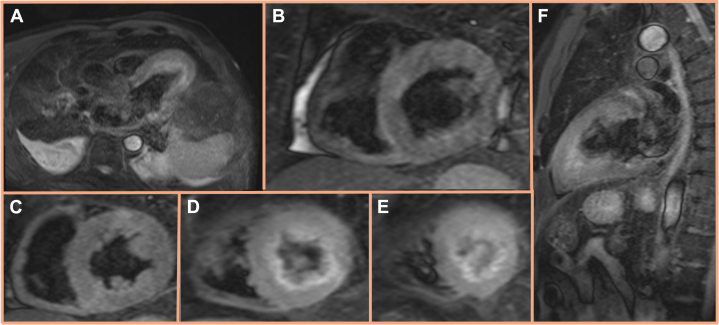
Cardiac Magnetic Resonance Findings Consistent With Stress Cardiomyopathy (A) T2-weighted short tau inversion recovery (STIR) short-axis view demonstrating increased myocardial signal intensity predominantly in the mid-to-apical left ventricular segments, consistent with myocardial edema extending beyond a single coronary territory. (B to E) T2-weighted STIR serial short-axis views from basal to apical segments demonstrating myocardial edema involving the mid and apical segments with apical predominance and relative basal sparing, extending beyond a single coronary territory, consistent with stress cardiomyopathy. (F) Late gadolinium enhancement phase-sensitive inversion recovery long-axis view demonstrating the absence of myocardial enhancement.
